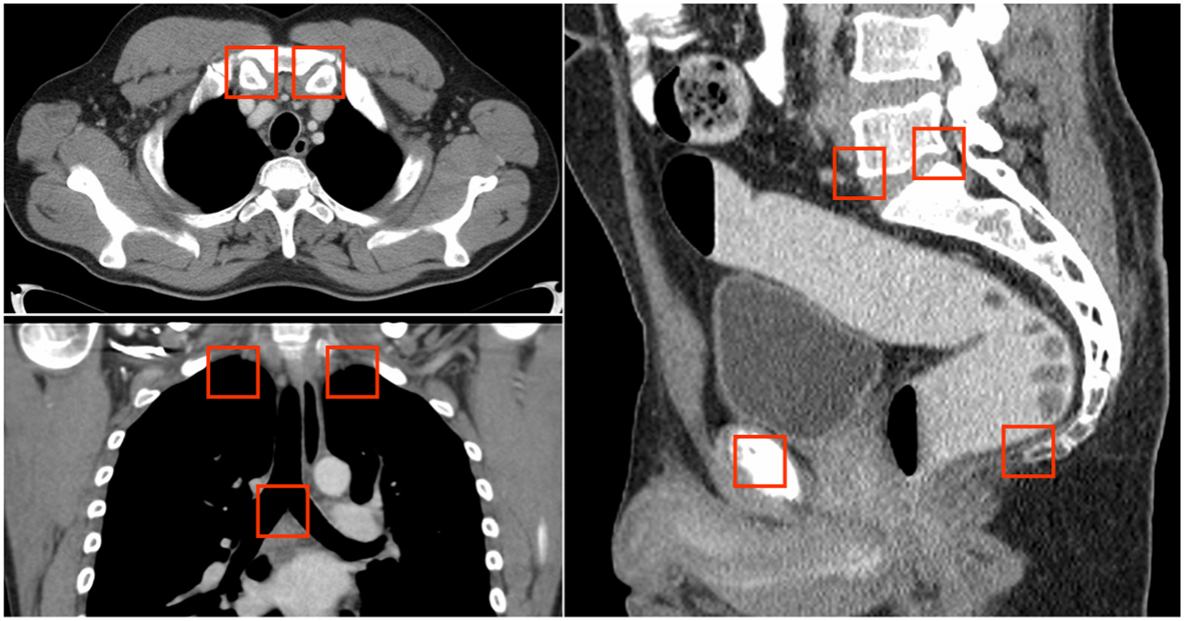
Detected CT landmarks. Examples of automatically detected anatomical landmarks in a thoraco/abdominal/pelvic CT-scan (inner tips of left and right clavicle, top of right and left lung, bronchial bifurcation, front and back of bottom plate of lumbar vertebra 5, pubic symphysis and coccygeal bone). Automatic detection of 44 thoracic, abdominal and pelvic landmarks in each CT-scan enables setting up a patient specific coordinate-system, cross-linkage of the coordinate-systems of consecutive CT-scans and a software-based navigation between baseline and follow-up CT-scans.
